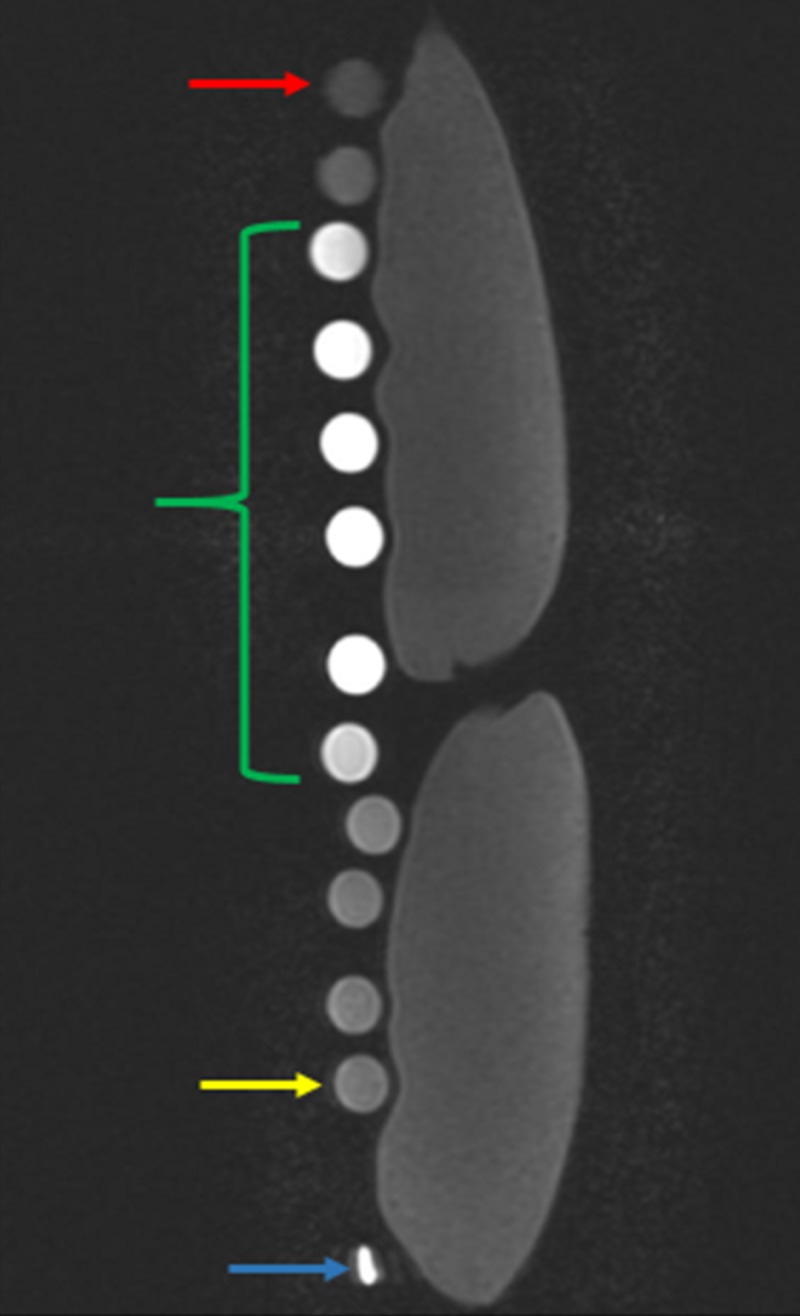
In vitro results of serial diluted gadolinium solution on T1 weighted magnetic resonance imaging The results of the in vitro experiment emulates the Bleicher and Kanal bell-shaped curve, where the test tube with the most and least concentrated gadolinium solutions are represented with the lowest signal intensity at the top (red arrow) and bottom (yellow arrow), respectively. The test tubes with gadolinium solution concentrations suitable for arthrographic joint injections lie within these concentration extremes (green bracket). All test tube samples were imaged adjacent to two 500 milliliter bags of normal saline. The blue arrow represents the magnetic resonance imaging marker.
